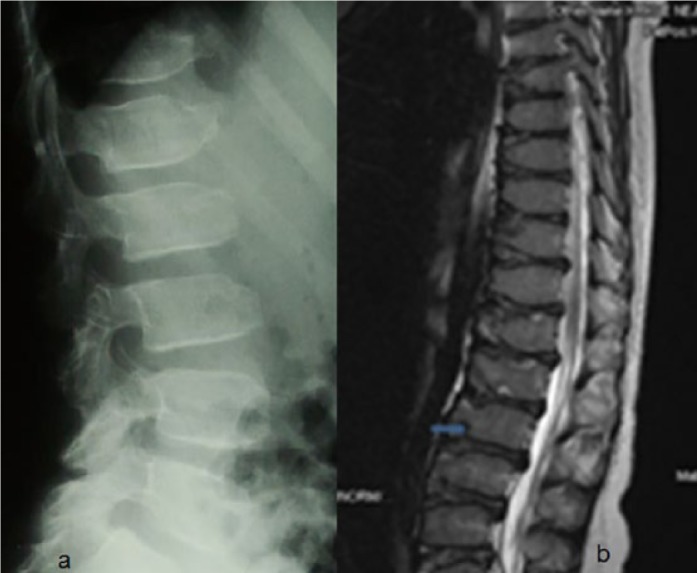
(a) Lateral lumbar spine radiograph in a 9-year-old girl showed defective ossification of the anterior portions of the upper and lower end-plates, and L-3 specifically showed marked anterior ossification defect (anteriorly pointing) associated with reduction of the height of the upper lumbar bodies. (b) Sagittal MRI of the lumbar spine of the same girl at the age of 13 years showed progressive platyspondyly, Schmorl’s node (arrow), anterior end-plates defective ossifications, and signs of osteochondritis (severe irregularities at the end plates of vertebral bodies with intervertebral and retromarginal herniations).
